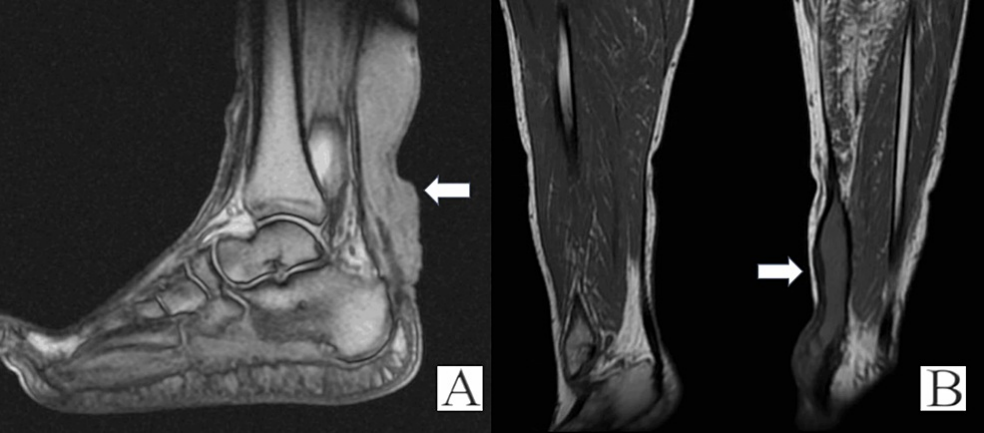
(A-B) T2 weighted sagittal MRI showing distinct masses in Achilles tendon
